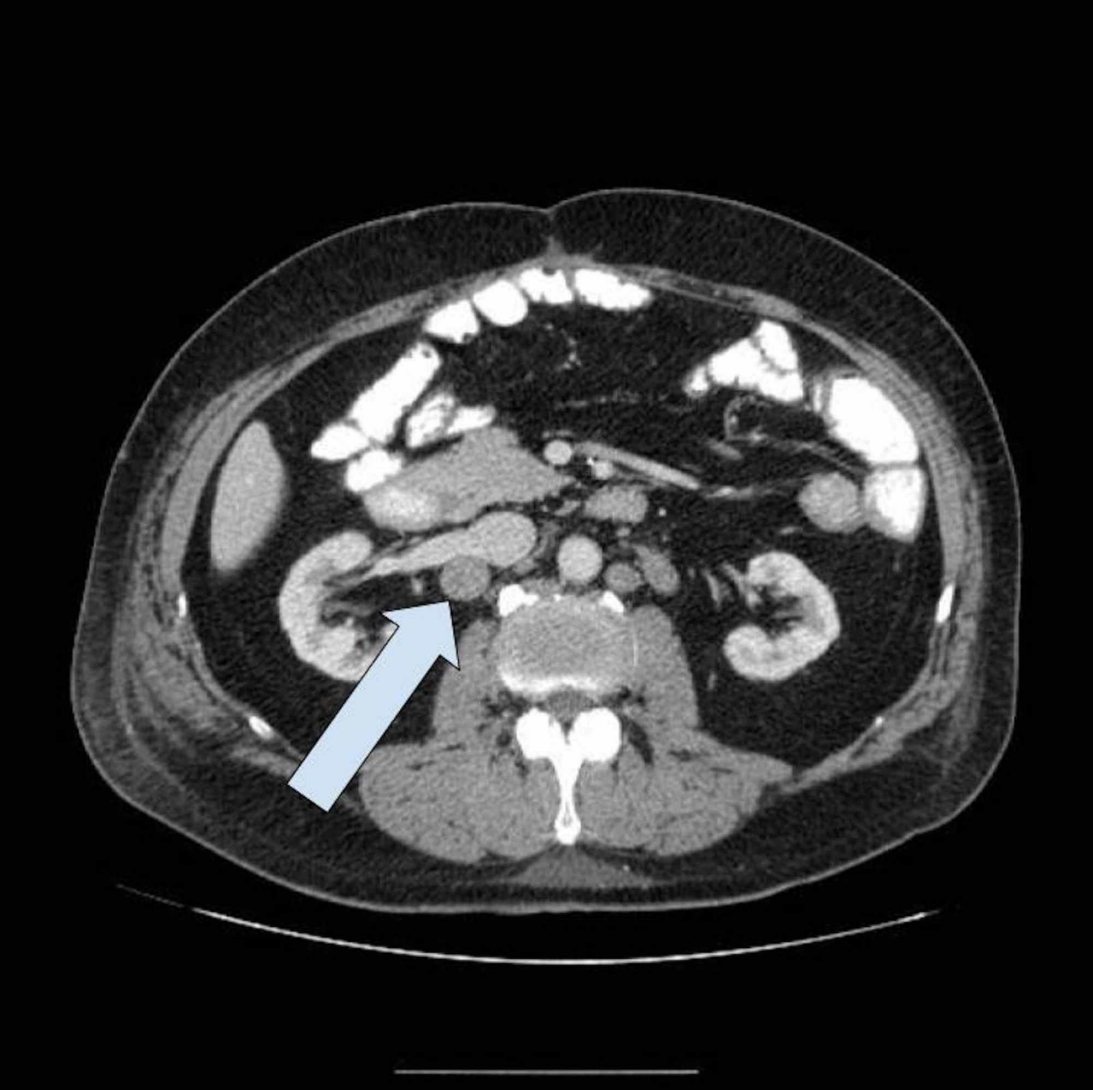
CT Abdomen and Pelvis with IV Contrast Interval worsening of retroperitoneal lymphadenopathy, representing metastatic rectal cancer.
